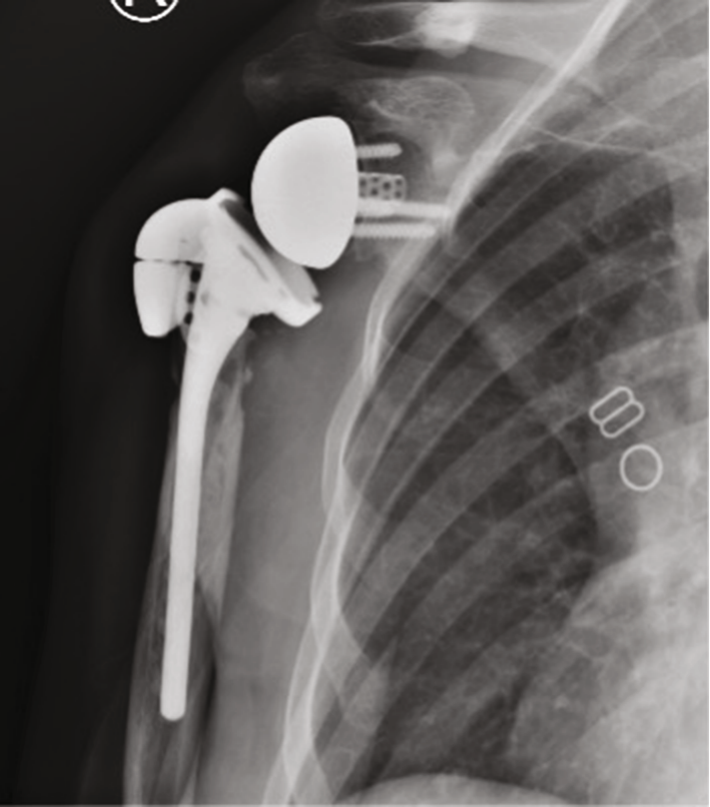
A 1-month follow-up AP radiograph after revision rTSA demonstrating intact hardware and stable prosthetic components.
